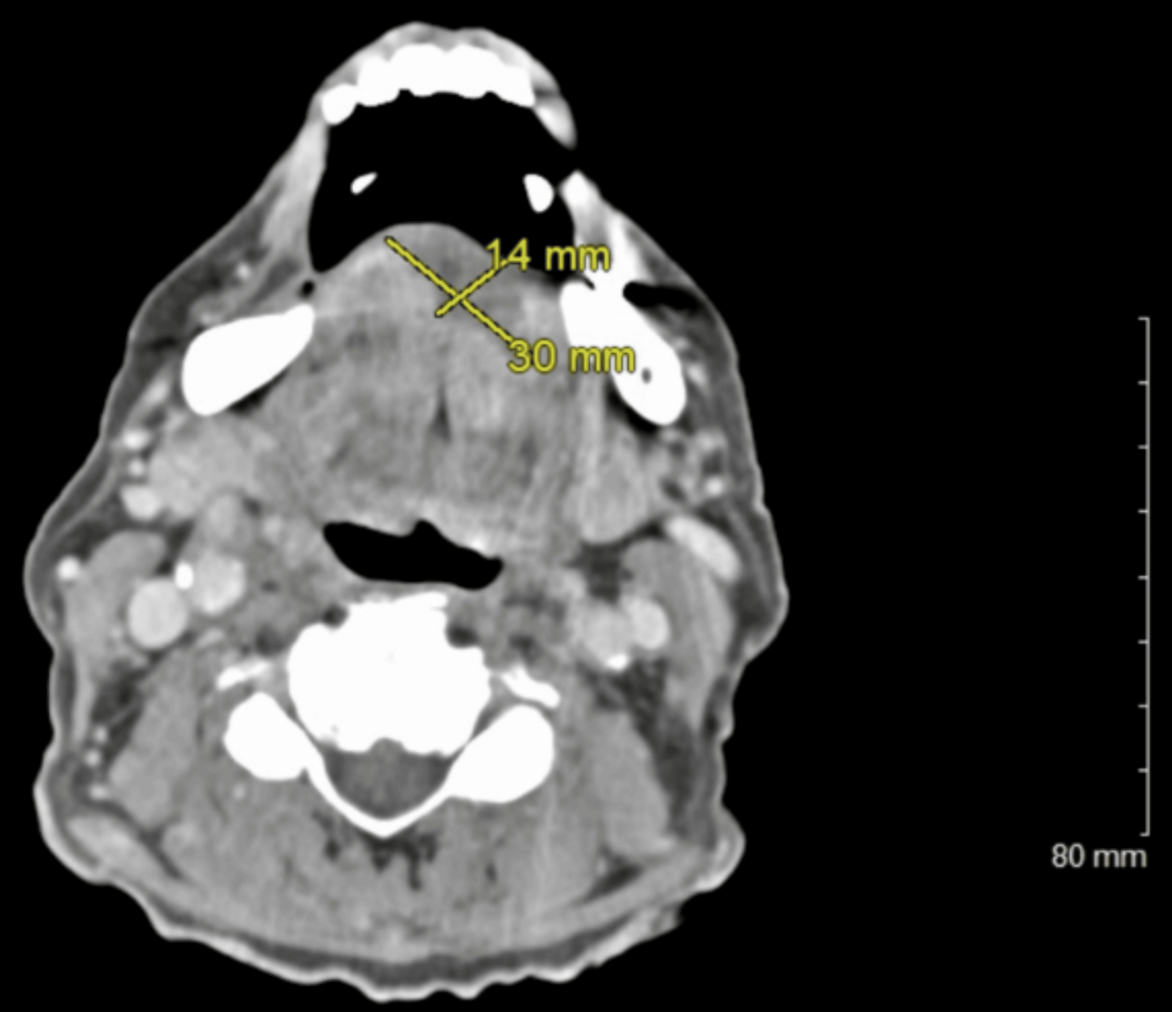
CT soft tissue neck, revealing that the lesion was 1.4 cm in height and 3.0 cm in length and that it extended down to about the floor of the mouth CT: computed tomography
